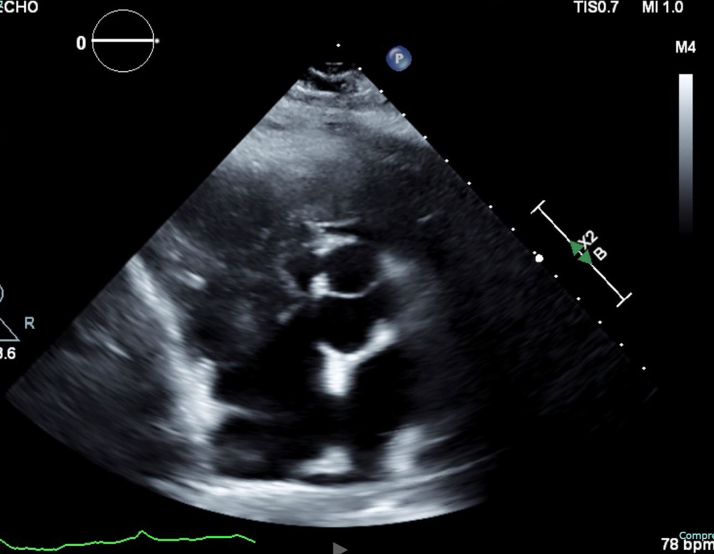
Transthoracic Echocardiogram of Subcostal Modified Short Axis Demonstrating a Large, Immobile Right Ventricular Mass Extending Into the Right Ventricular Cavity The mass appears attached to the anterior RV wall and contributes to right ventricular outflow tract (RVOT) obstruction.
